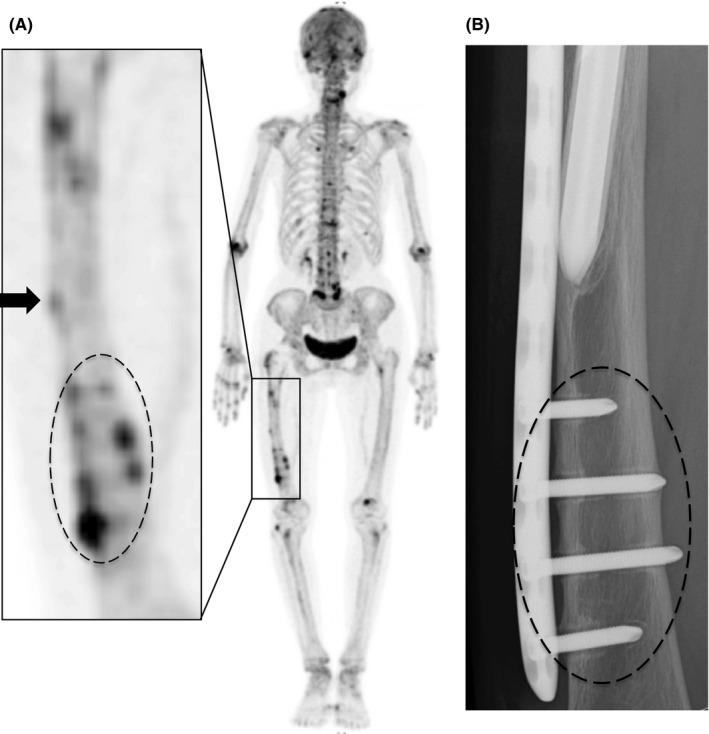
A, ^18^F‐sodium fluoride positron emission tomography (NaF PET) image obtained 1.5 y following surgery showed that the high NaF uptake at the beaking of the lateral cortex a little above the stem tip was dramatically reduced (black arrow). There was high‐intensity area around the distal screws below the stem end (dotted circle) A higher magnification image of the framed area is shown in the left panel. B, Radiograph of the right femur (anteroposterior) taken at the same time with the NaF PET/CT scan showed radiolucent zones in the corresponding area of the NaF PET image. (dotted circle)
